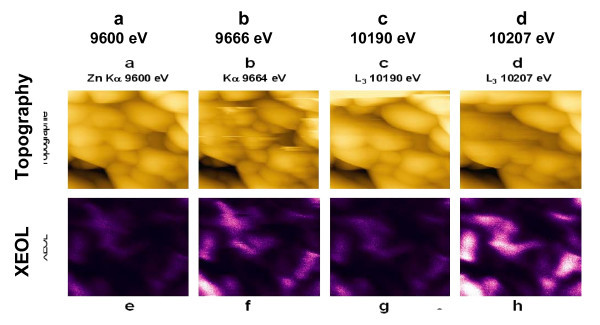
Twin topography-luminescence images. Top: (a-d) topography of a ZnO-ZnWO_4_ sputtered layer (2 × 2 μm^2^). Bottom: corresponding visible light emission cartography under illumination by X-ray beam from left to right below (e) and above (f) the Zn-Kα threshold (9.6 keV) and below (g) and above (h) the W-L_3_threshold (10.2 keV). On top of the images is indicated the X-ray primary energy.
